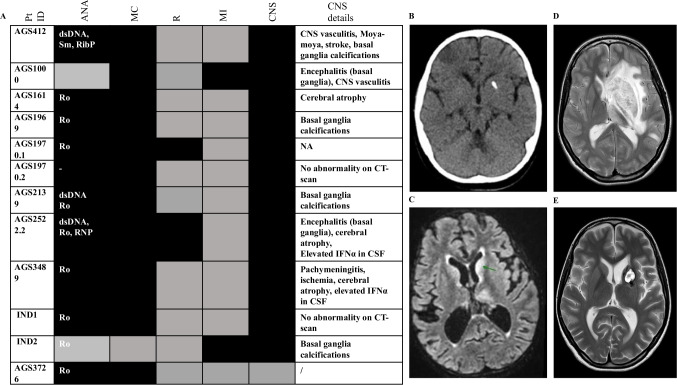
Clinical Features of patients with C1QDef in our cohort. A For each patient, presence (dark) or absence (grey) of the following features is indicated: Anti-nuclear antibody (ANA- specific antibodies are written in the cell if present), mucocutaneous (MC), renal (R), major infection (MI) or central nervous system (CNS) involvement. Black and grey boxes indicate, respectively, the presence or absence of disease. Details of CNS involvement are given in the last column. B CT-scan of patient AGS412 showing basal ganglia calcification. C MRI of patient AGS2522.2 showing encephalitis with signal abnormalities in the basal ganglia and thalami (MRI sequence T2 FLAIR). D MRI (T2W) of patient AGS1000 at relapse of CNS inflammation, showing diffuse enlargement of the left basal ganglia, caudate nucleus and thalamus, with mass effect. E MRI (T2W) of patient AGS1000 two months after (D), showing significant reduction in the size of the lesion and perilesional oedema, and post biopsy changes
